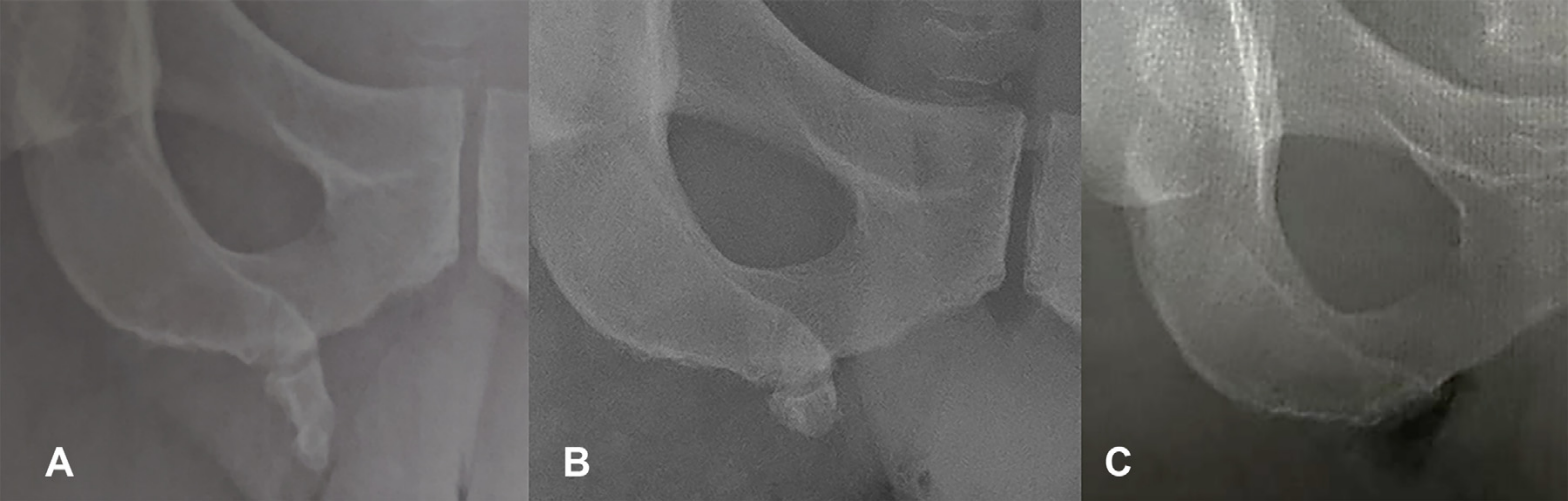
Pelvic radiographs (frontal view) before (A) and after (B) partial surgical resection of the pelvic digit. Complete resection was performed 9 months later (C).
